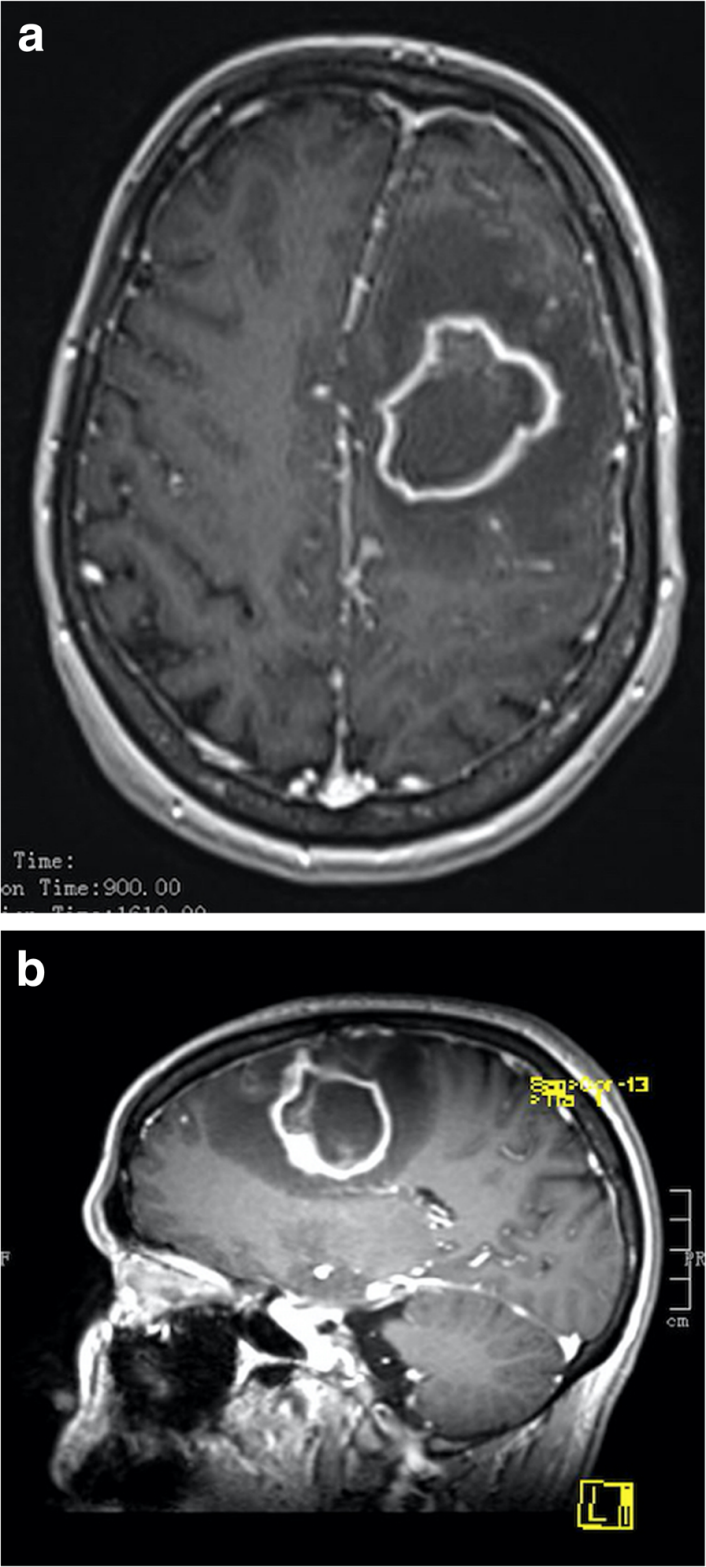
T1-weighted contrast-enhanced horizontal (a) and sagittal (b) MRI image of the abscess-like cerebral lesion. Ring-enhancing lesion with a central low intensity content and peripheral low intensity, the latter of which is due to the surrounding extensive vasogenic edema
